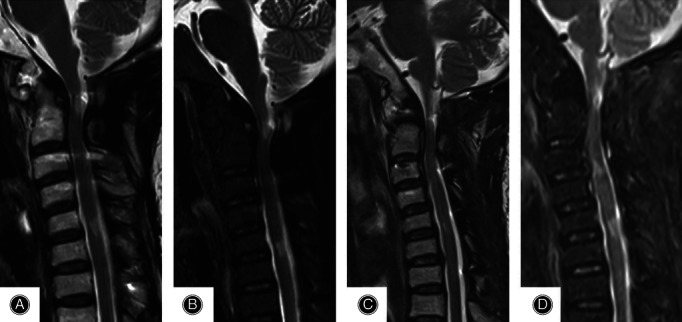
(A, B) MRI showed that the compression of spinal cord was resolved half one year after surgery. (C, D) MRI showed that the spinal cord remained uncompressed and the patient achieved perfect surgical results one year after surgery.
